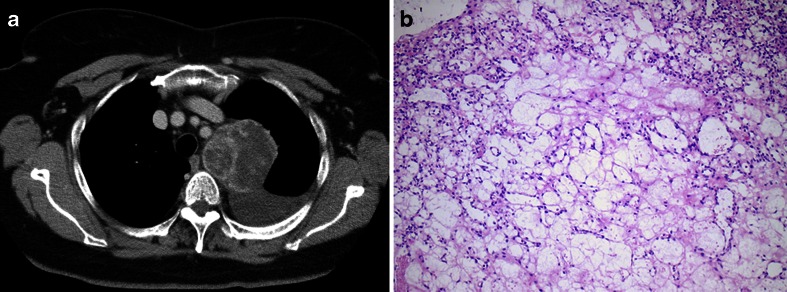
Angiolipoma (lipomatous hemangioma). Tumour in the superior mediastinum in a 67-year-old female who had few complaints but had persistent pleural effusion. The mass was excised, and no recurrence was noted after several years of follow-up. a Axial contrast-enhanced CT image revealing a circumscribed mediastinal mass with variable density. The mass was found to have been present for several years on retrospective evaluation of previous examinations. b Mature fat admixed with thin-walled vessels; there was no atypia of endothelial cells
